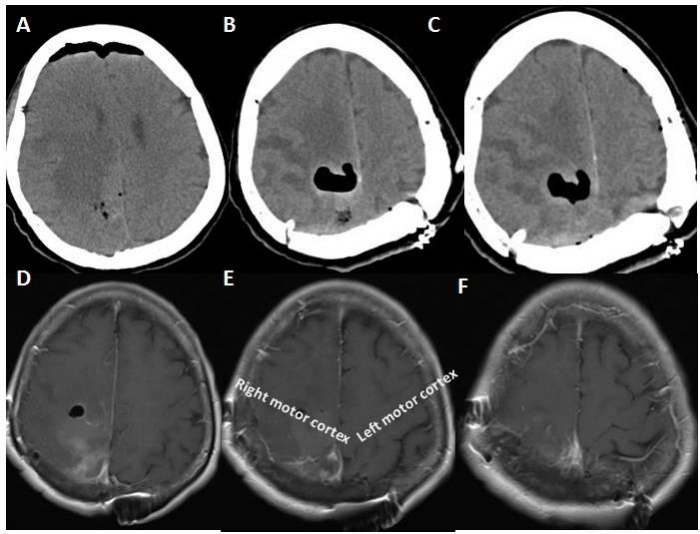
A, B, C: (Table 1: Case 1, Grade 2 meningioma): Noncontrasted cranial computerized tomography was taken 24 hours after the operation. It shows no residual tumour and hemorrhage at the operation site being right parietal and posterior frontal lobe region. D, E, F: T1 weighted gadolinium-enhanced axial magnetic resonance imaging (MRI) scans showing right frontoparietal operation region and hypointense oval- shaped area at the right motor cortex. And there is no residual tumour this region*.
